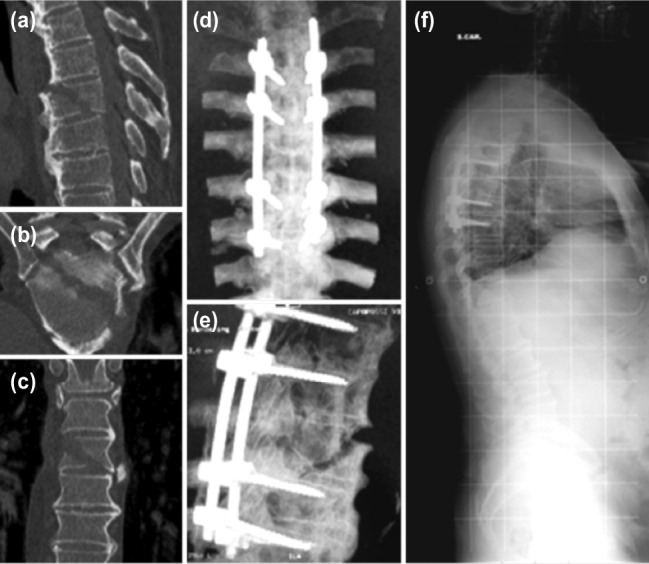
(a,b,c) CT-scan investigation in a male patient affected by ankylosing spondylitis, revealing severe T6 fracture starting from the ossified anterior longitudinal ligament and involving the disc and the vertebra. (d,e) Postoperative CT-scan 3D reconstruction showing the correct placement of the screws in T4, T5, T7 and T8. (f) Radiograph of the spine at two years follow-up, no movement or implant loosening evident.
